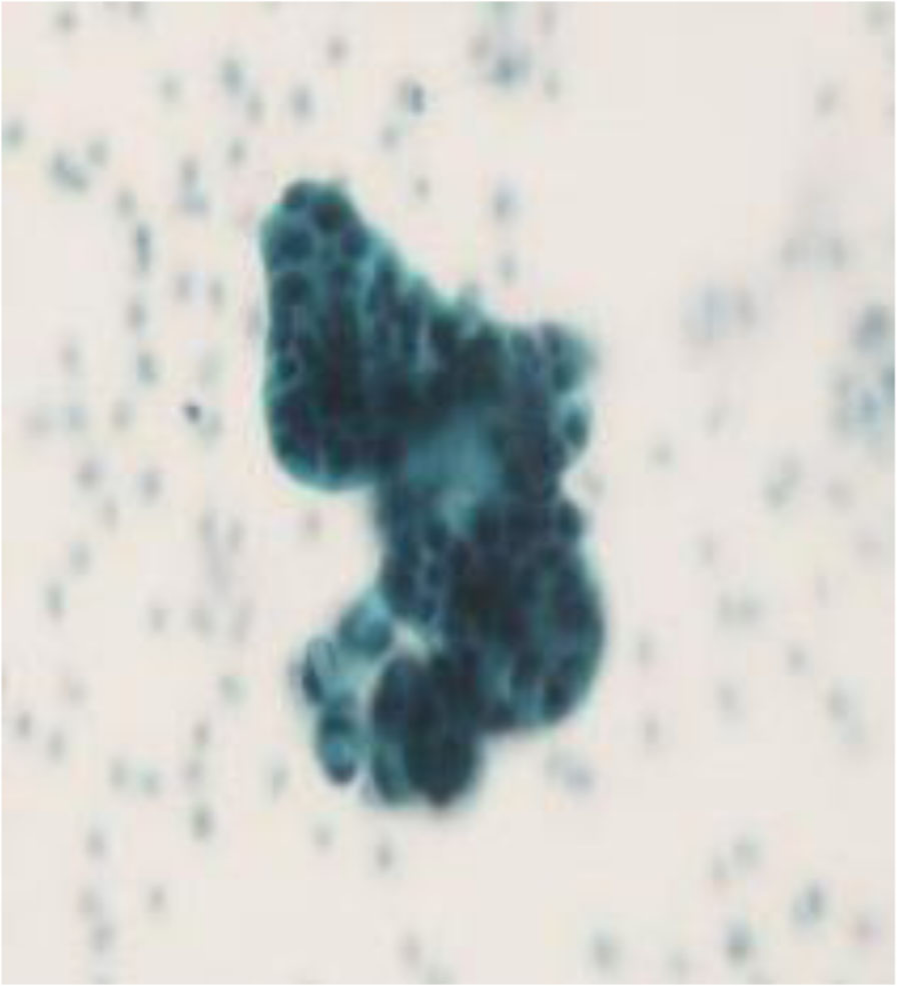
Abdominal magnetic resonance imaging demonstrating T2 high and a fluid-fluid level
